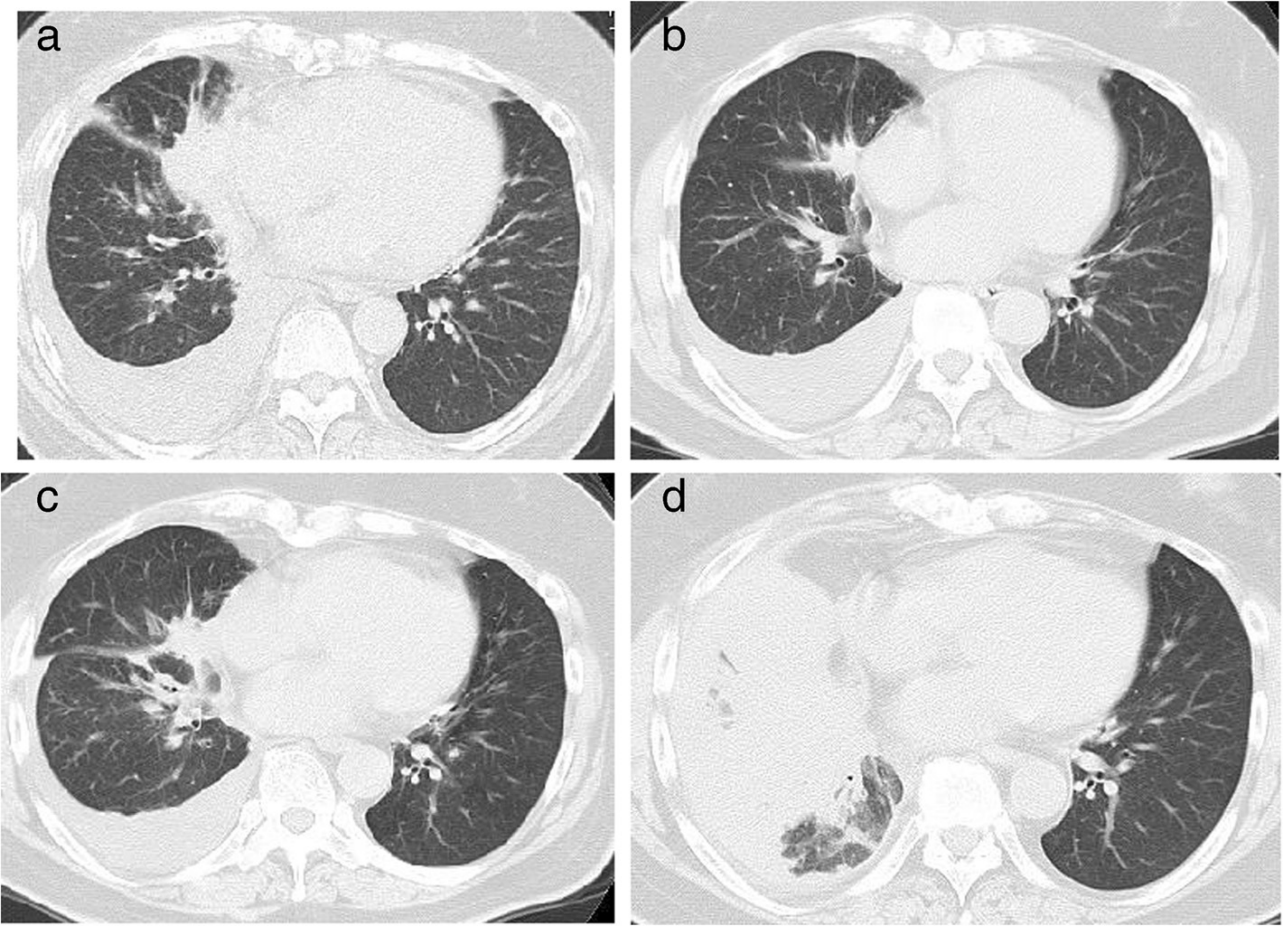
Computed tomography scans. a) On the initial medical examination, right pleural effusion and a mass in the middle lung field were observed. b) After 3 months of gefitinib treatment, an improvement in the right pleural effusion and a decrease in the size of the primary lesion were observed. c) After 5 months of gefitinib treatment, progression of the size of the mass was observed. d) Infiltration and atelectasis of the right lung were observed.
